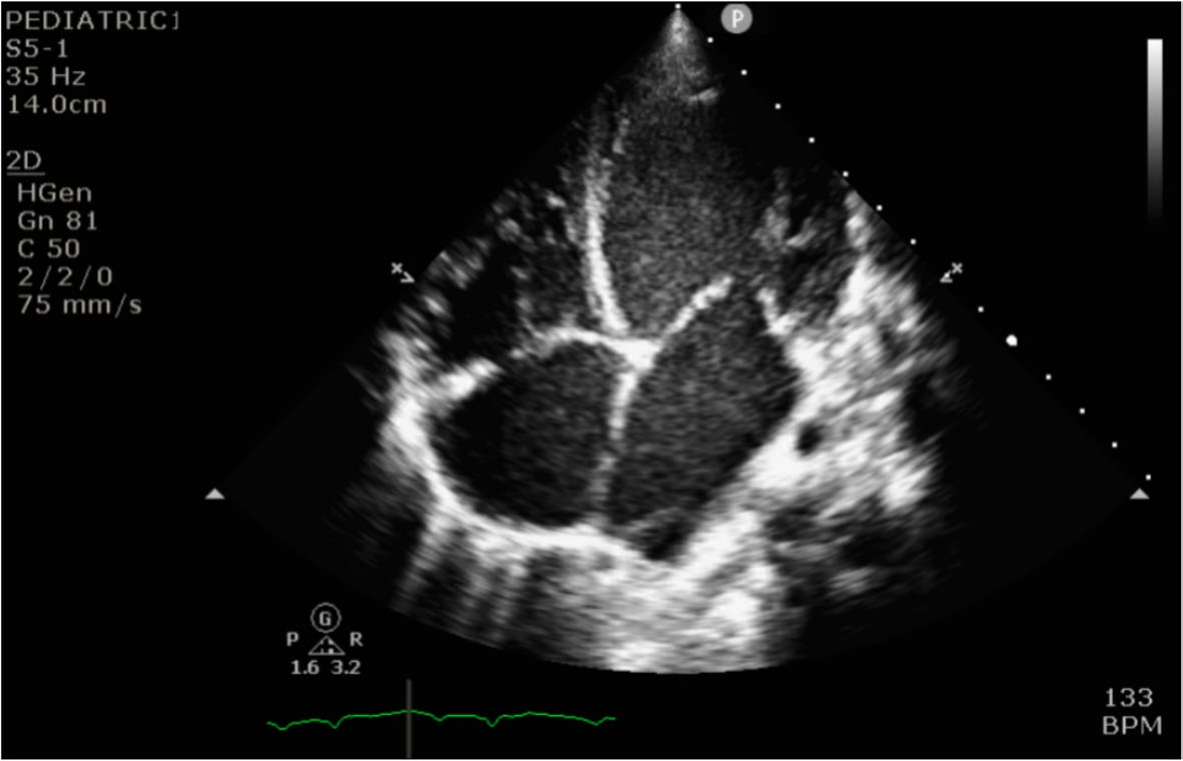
Echocardiogram upon admission showing dilated left ventricle
